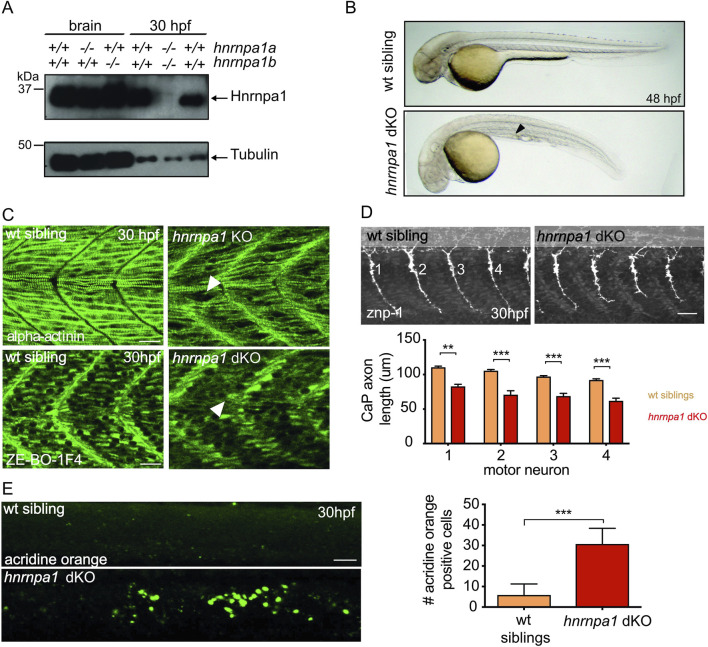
Hnrnpa1 dKO show a lipid, muscle, motor neuron phenotype and increased cell death (A) The antibody HA1-CT (VM) AN351 detects Hnrnpa1a and Hnrnpa1b since a band at the expected size of Hnrnpa1a and Hnrnpa1b is detectable in wildtype, *hnrnpa1a*
